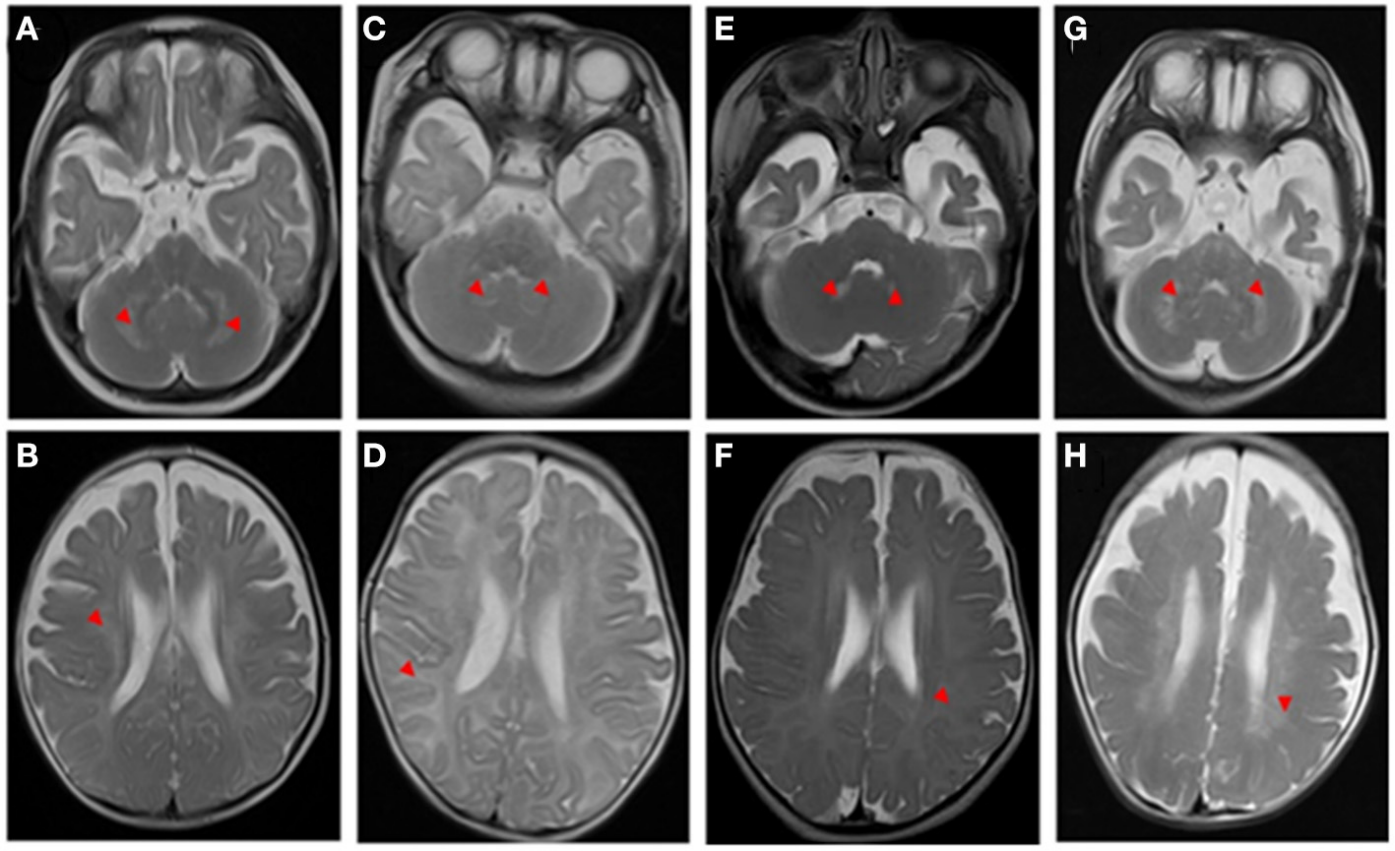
T2-weighted brain magnetic resonance imaging (MRI) of the patients. The red arrowheads show the lesions. Neuroimaging findings (A,B, case 1)at the 5-month-old show a cerebellar dentate nucleus and white matter were damaged, and the subcortical U-shaped fibers were significantly involved; (C,D, case 2) at a 3-month-old show diffuse lesions in white matter, obvious subcortical involvement of U-shaped fibers, and suspicious lesions in cerebellar dentate nuclei; (E, F, case 3) at a 3-month-old show suspicious lesions in the cerebellar dentate nucleus and the change of cerebral white matter is not prominent; (G,H) at the 6-month-old evident lesions in the cerebellar dentate nucleus.
